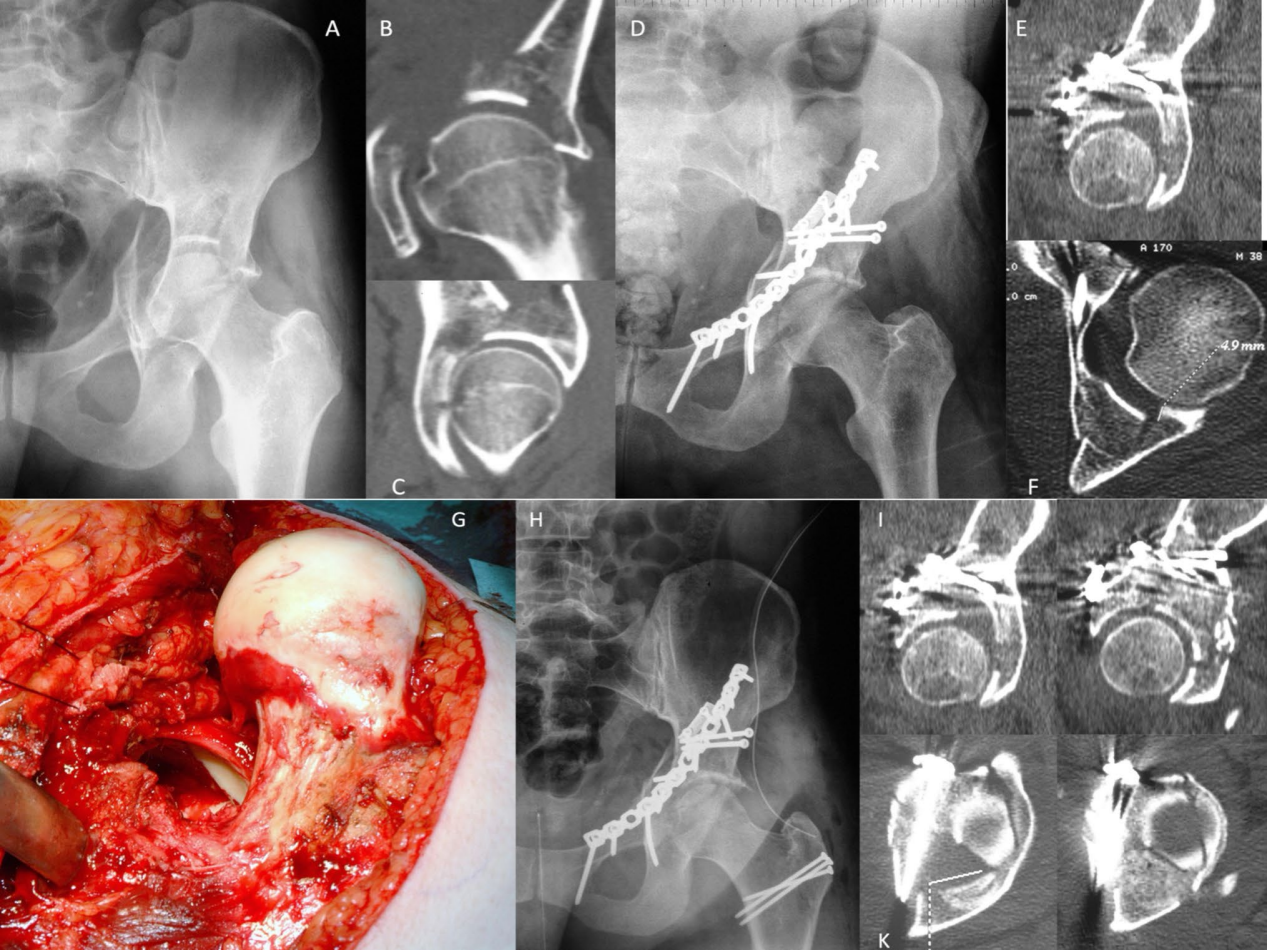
35 year old male sustaining a simple fall on the left lateral hip. Imaging shows an anterior column fracture (A) with marginal impactions cranially (B) and posteriorly, while the posterior column was intact (C). Open reduction and internal fixation was performed via an ilioinguinal approach with adequate reconstruction in the anterior part of the acetabulum (D). A postoperative CT scan shows that the posterior marginal impaction was not addressed (E, F). Thus, additional open reduction was performed via a Kocher-Langenbeck approach with surgical hip dislocation (G) resulting in adequate reconstruction of the articular surface (H). Comparison of preoperative and postoperative CT scans (I, K)
